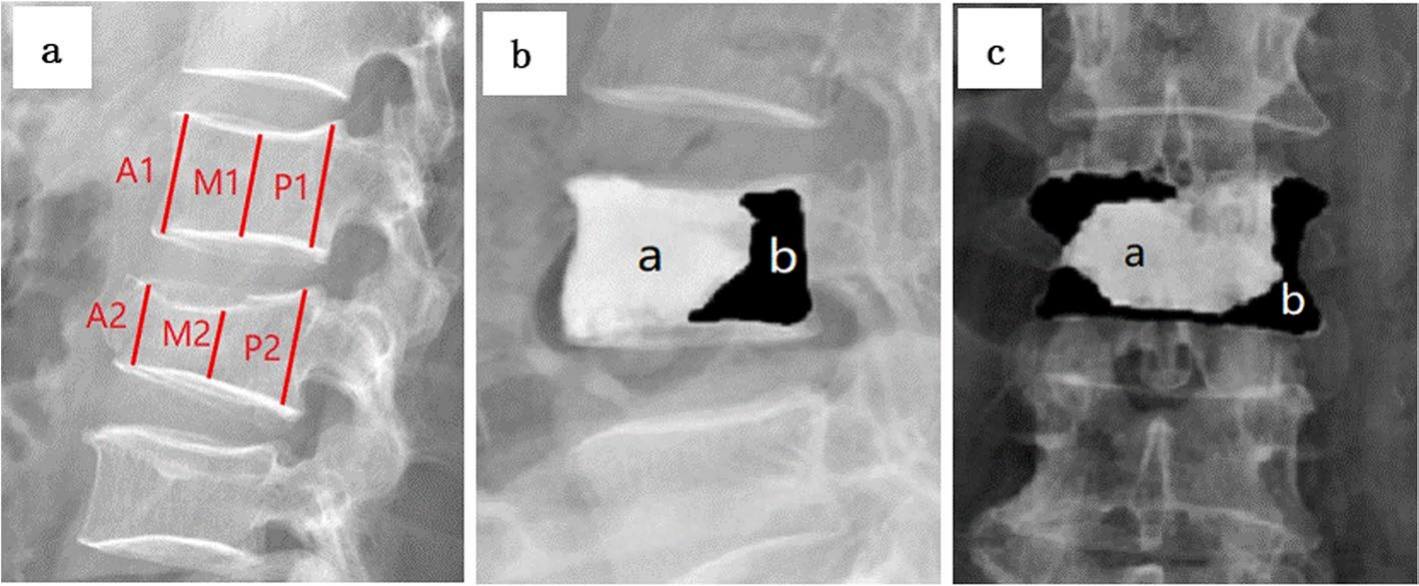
a-c Methods of measurements on images. a Lateral radiograph showing evaluation of the anterior vertebral body height ratio (AVBHr), middle vertebral body height ratio (MVBHr), and posterior vertebral body height ratio (PVBHr). AVBHr=A2/A1, MVBHr=M2/M1, and PVBHr=P2/P1. b-c Lateral and Frontal radiograph showing the evaluation of cement distribution. Cement distribution =a/(a+b)
